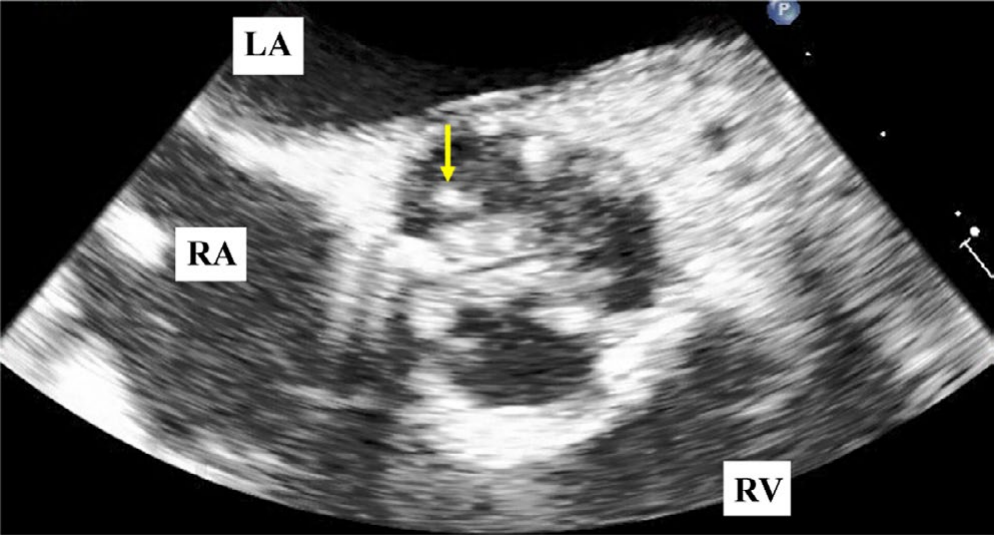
A polypoid lesion on the non‐coronary cusp (yellow arrow) with a calcified aortic valve on transesophageal echocardiography. LA, left atrium; RA, right atrium; RV, right ventricle
